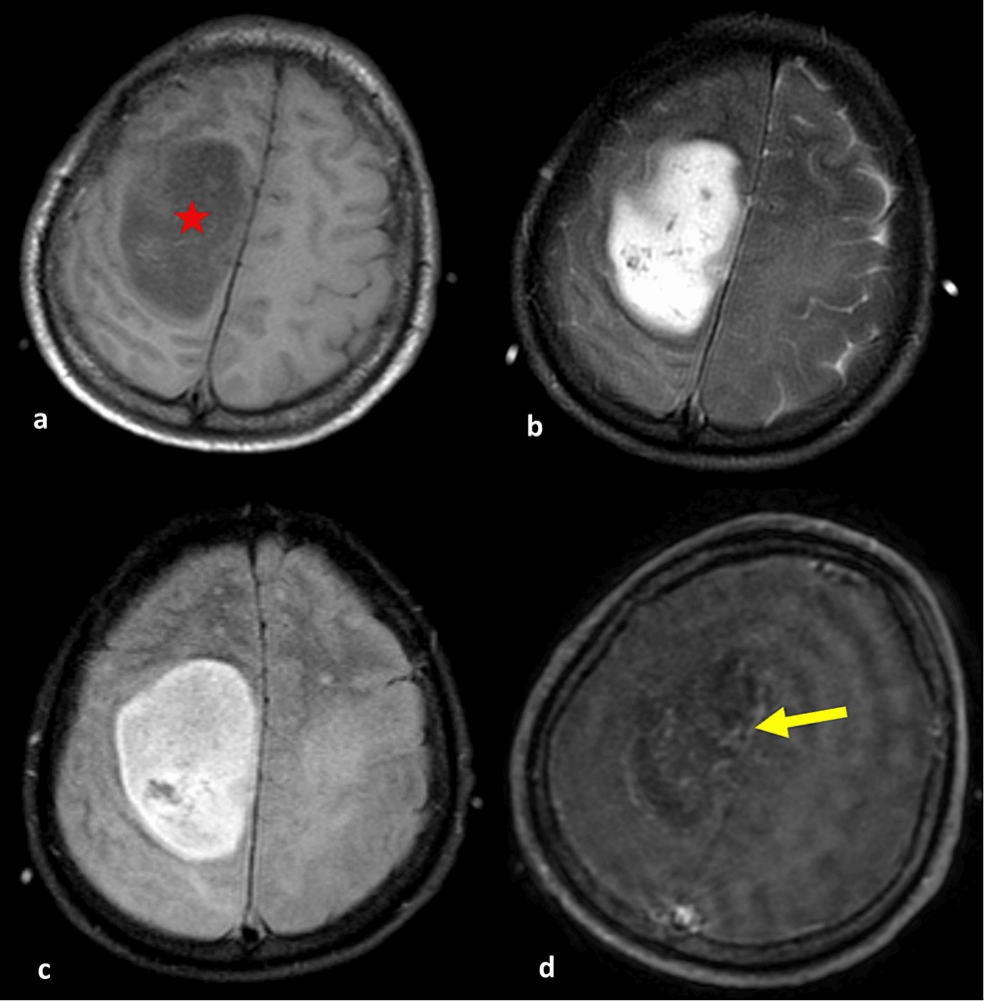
A 55-year-old male presenting with an intra-axial mass. (a) Axial T1-weighted, (b) T2-weighted, (c) FLAIR, and (d) post-contrast T1-weighted MR images showed the tumor is located in the right frontal lobe (f1) (red star) and exhibits ill-defined boundaries in the enhancing tumor region (f12) (yellow arrow). There is an absence of perifocal edema, extensive necrotic areas, or nodular solid-enhancing regions. Although the morphological features of this lesion suggest a low-grade diffuse astrocytoma, molecular examination reveals an IDH-wildtype. Consequently, according to the WHO CNS 2021 classification, this tumor is categorized as an astrocytoma, IDH-wildtype, CNS WHO grade 4. IDH, isocitrate dehydrogenase; FLAIR, fluid-attenuated inversion recovery; MR, magnetic resonance; WHO, World Health Organization; CNS, central nervous system
